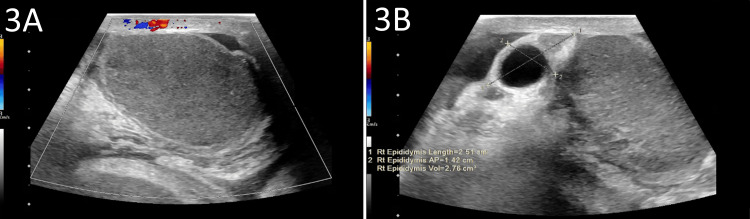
Ultrasound images of scrotum (A) The image demonstrates absent blood flow to the enlarged right testicle, measuring 4.2 x 2.8 x 2. 8 cm; (B) Ultrasound also reveals an enlarged right epididymis, measuring 2.5 x 1.4 cm.
